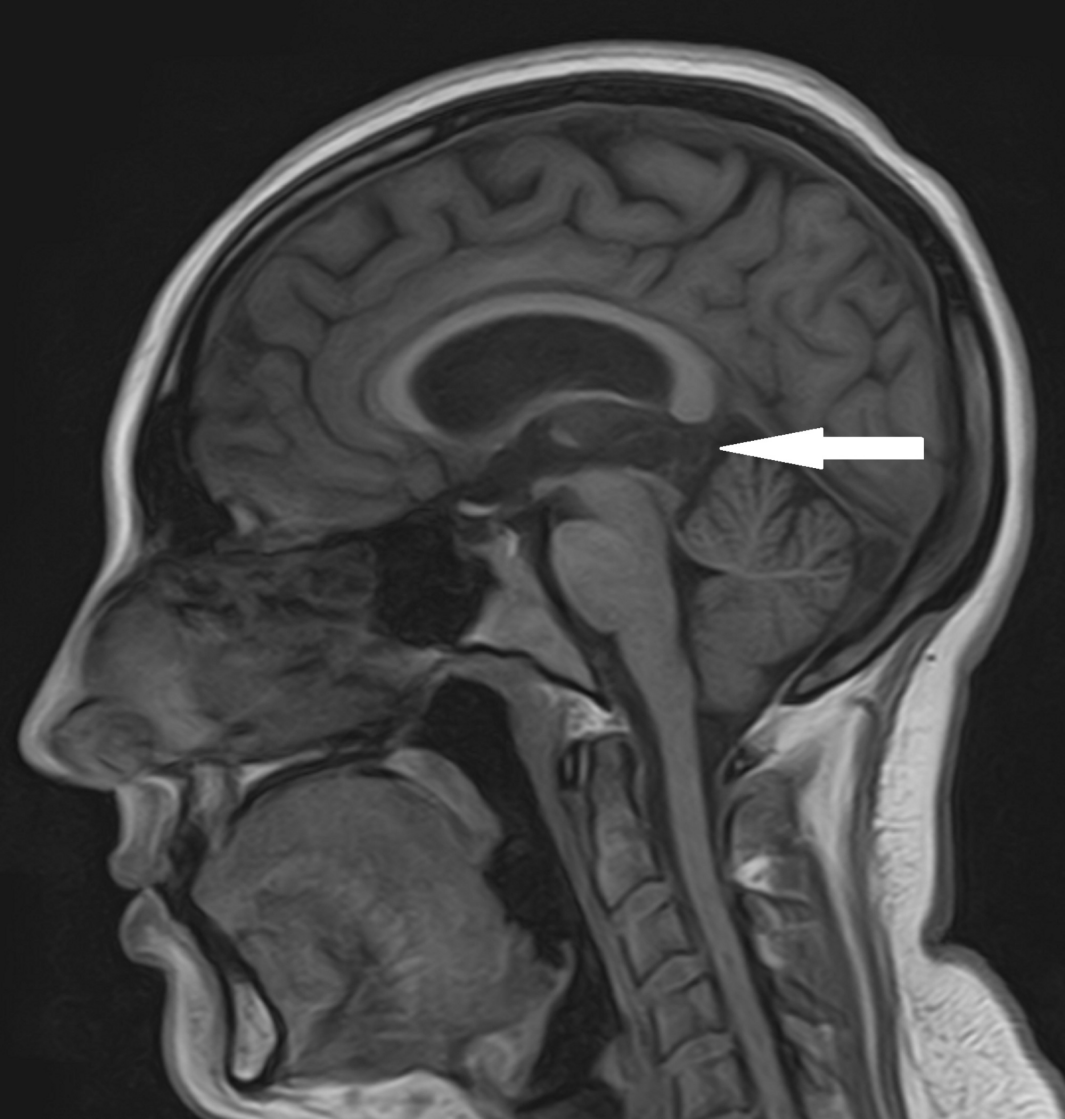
T1-weighted non-enhanced sagittal magnetic resonance imaging (MRI) demonstrating compression of the tectum by the cyst (highlighted by the white arrow).
